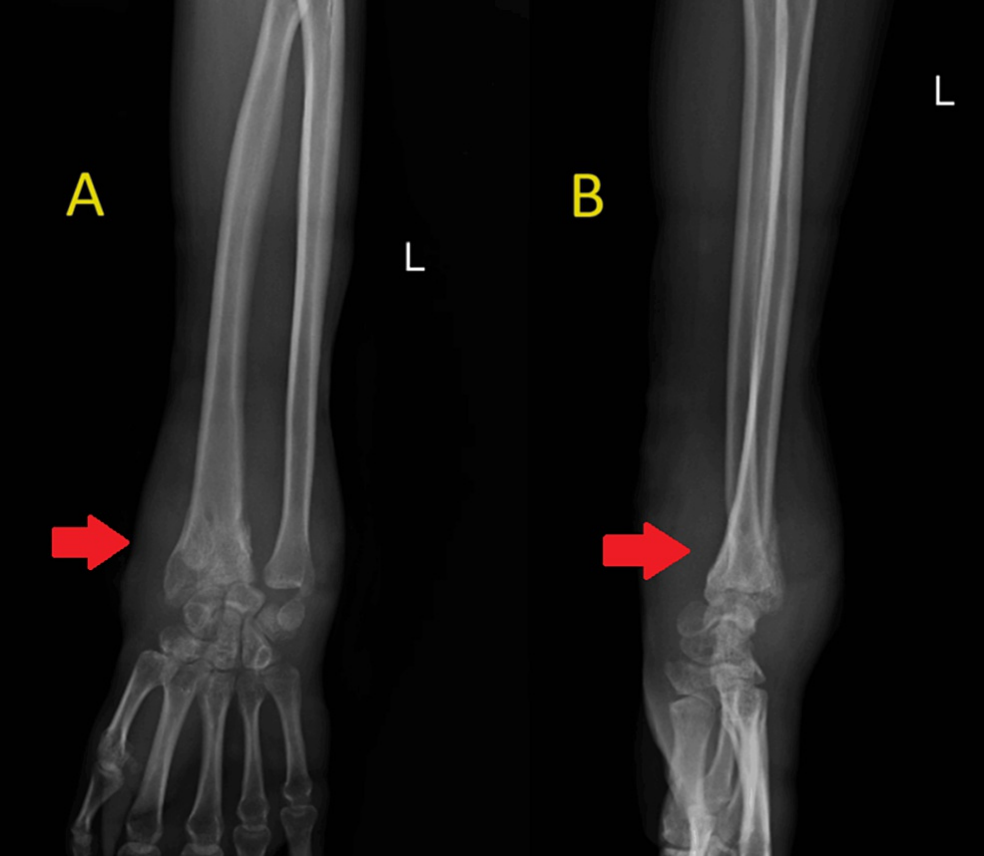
Post-operative X-ray of left wrist anteroposterior (A) and lateral (B) view after k wire removal, debridment, curettage, and sinus tract excision, showing no sign of infection
